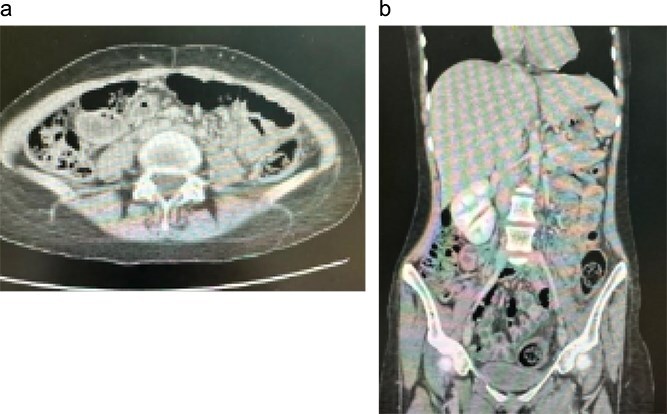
Follow-up contrast-enhanced CT abdomen and pelvis. (a) Axial image demonstrating resolution of the previously noted circumferential rectosigmoid wall thickening and pericolic inflammatory changes, with no residual pelvic mass or collection. (b) Coronal reformatted image confirming marked regression of rectosigmoid and peritoneal disease, consistent with a favorable radiologic response to prolonged voriconazole therapy.
